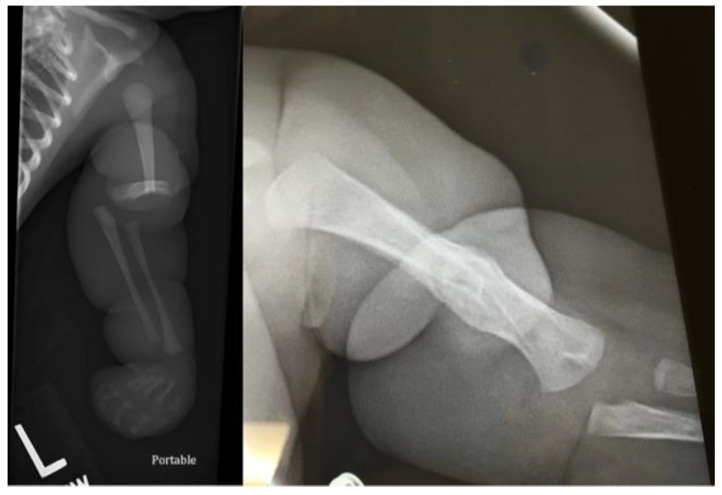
Radiographs of Patient 1: preoperative and 1-year postoperative with healed fracture (left to right).
